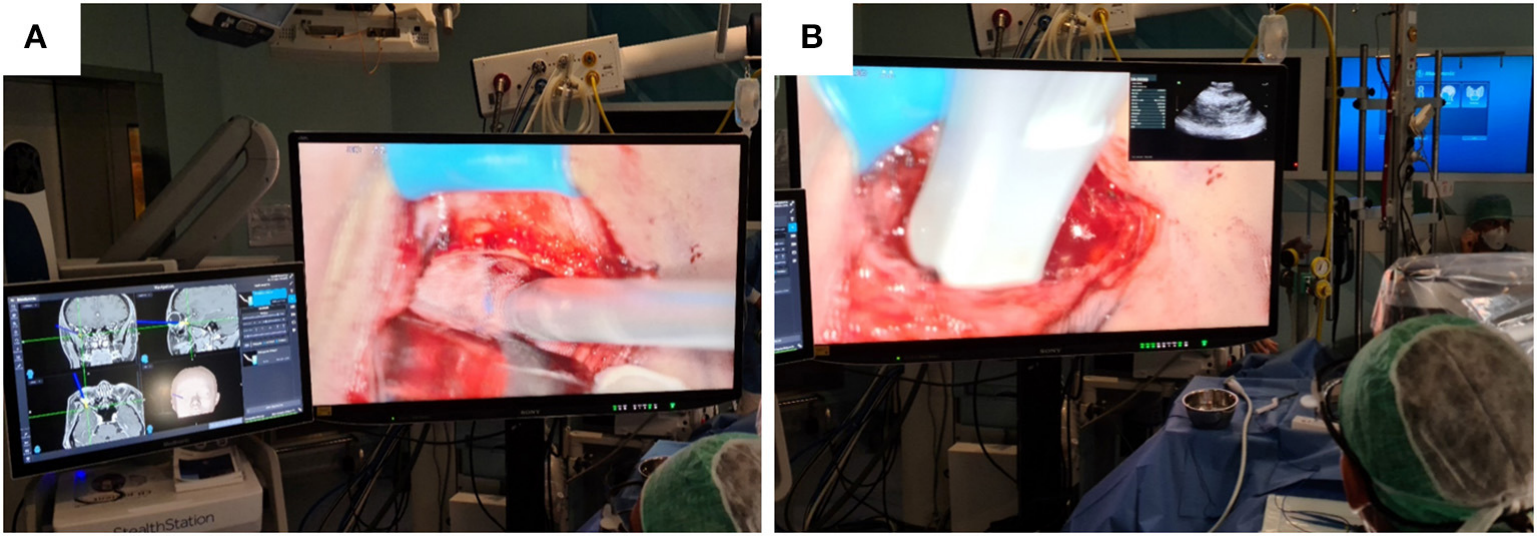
On the 55-inch monitor, the navigation device (A) and ultrasound probe (B) used for precise localization of the hemangioma inside the periorbita are clearly visible. The PiP mode (B) allows the surgeon to control the position of the ultrasound probe without having to look away from the operating field.
